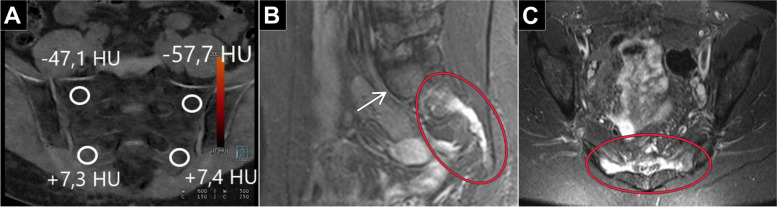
a Coronal VNCa reformatting of dual-energy CT suggested the absence of edema at the upper measurement sites (-47.1 HU and -57.7 HU), whereas the presence of edema was indicated for the lower measurement sites (+ 7.3 HU and + 7.4 HU). b Corresponding sagittal T2( STIR image (at 1.5 Tesla) of the same patient with marked edema in the lower sacrum (red circle). In contrast, no edema was ascertained in the upper sacrum (white arrow). c Axial T2 STIR image (at 1.5 Tesla) confirmed the presence of edema in both sides of the lower sacrum (red circle)
